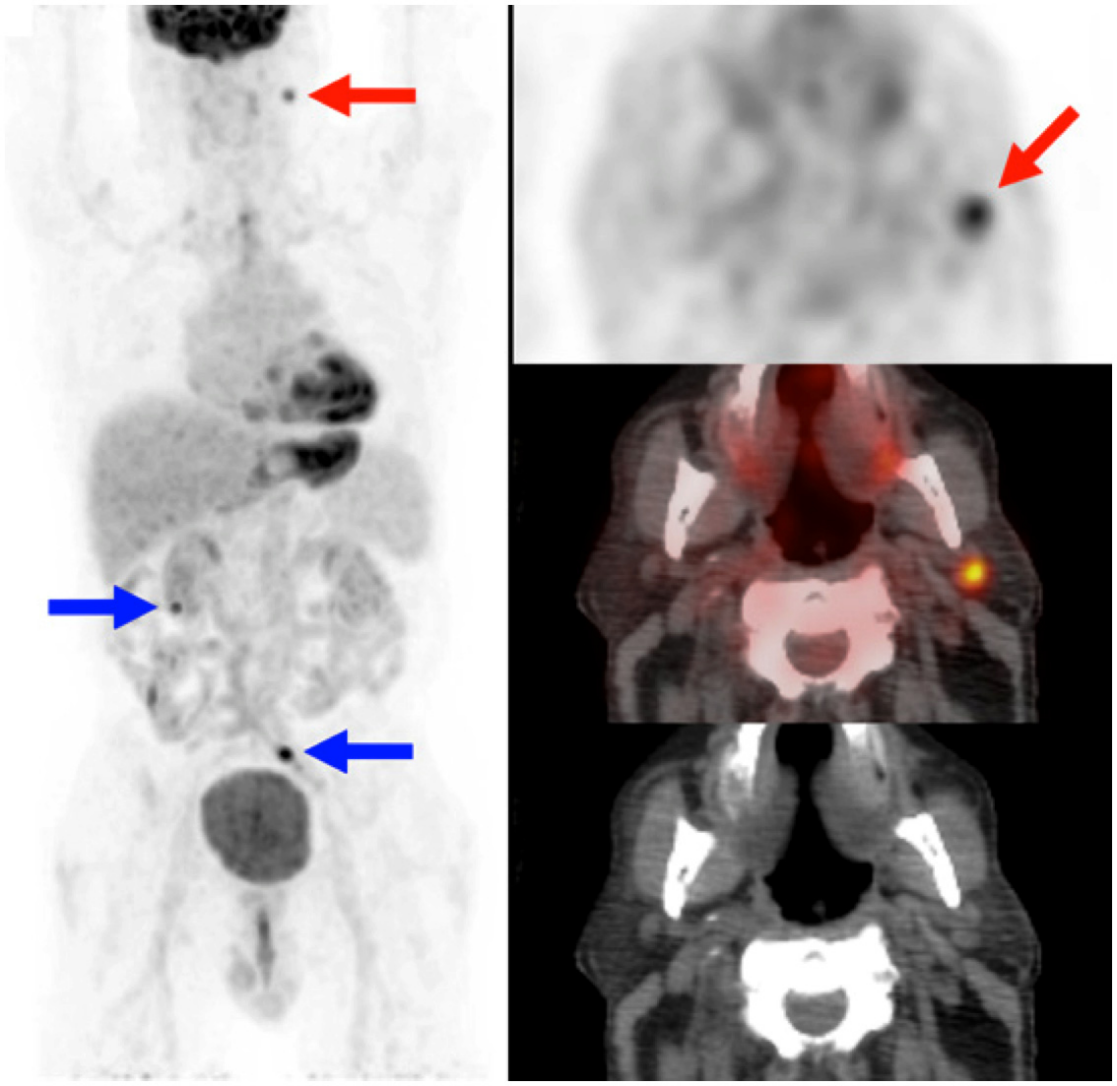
Example head/neck area. Significant false-positive finding on PET/CT in the head/neck area. Intense FDG uptake in a lymph node (red arrow), revealing inflammation in a patient who eventually did not have lung cancer. Bowel-hotspots were ignored (blue arrows). Left: maximum intensity projection of PET. Right: Corresponding transverse slices of PET (top), PET/CT (middle), and CT (bottom).
